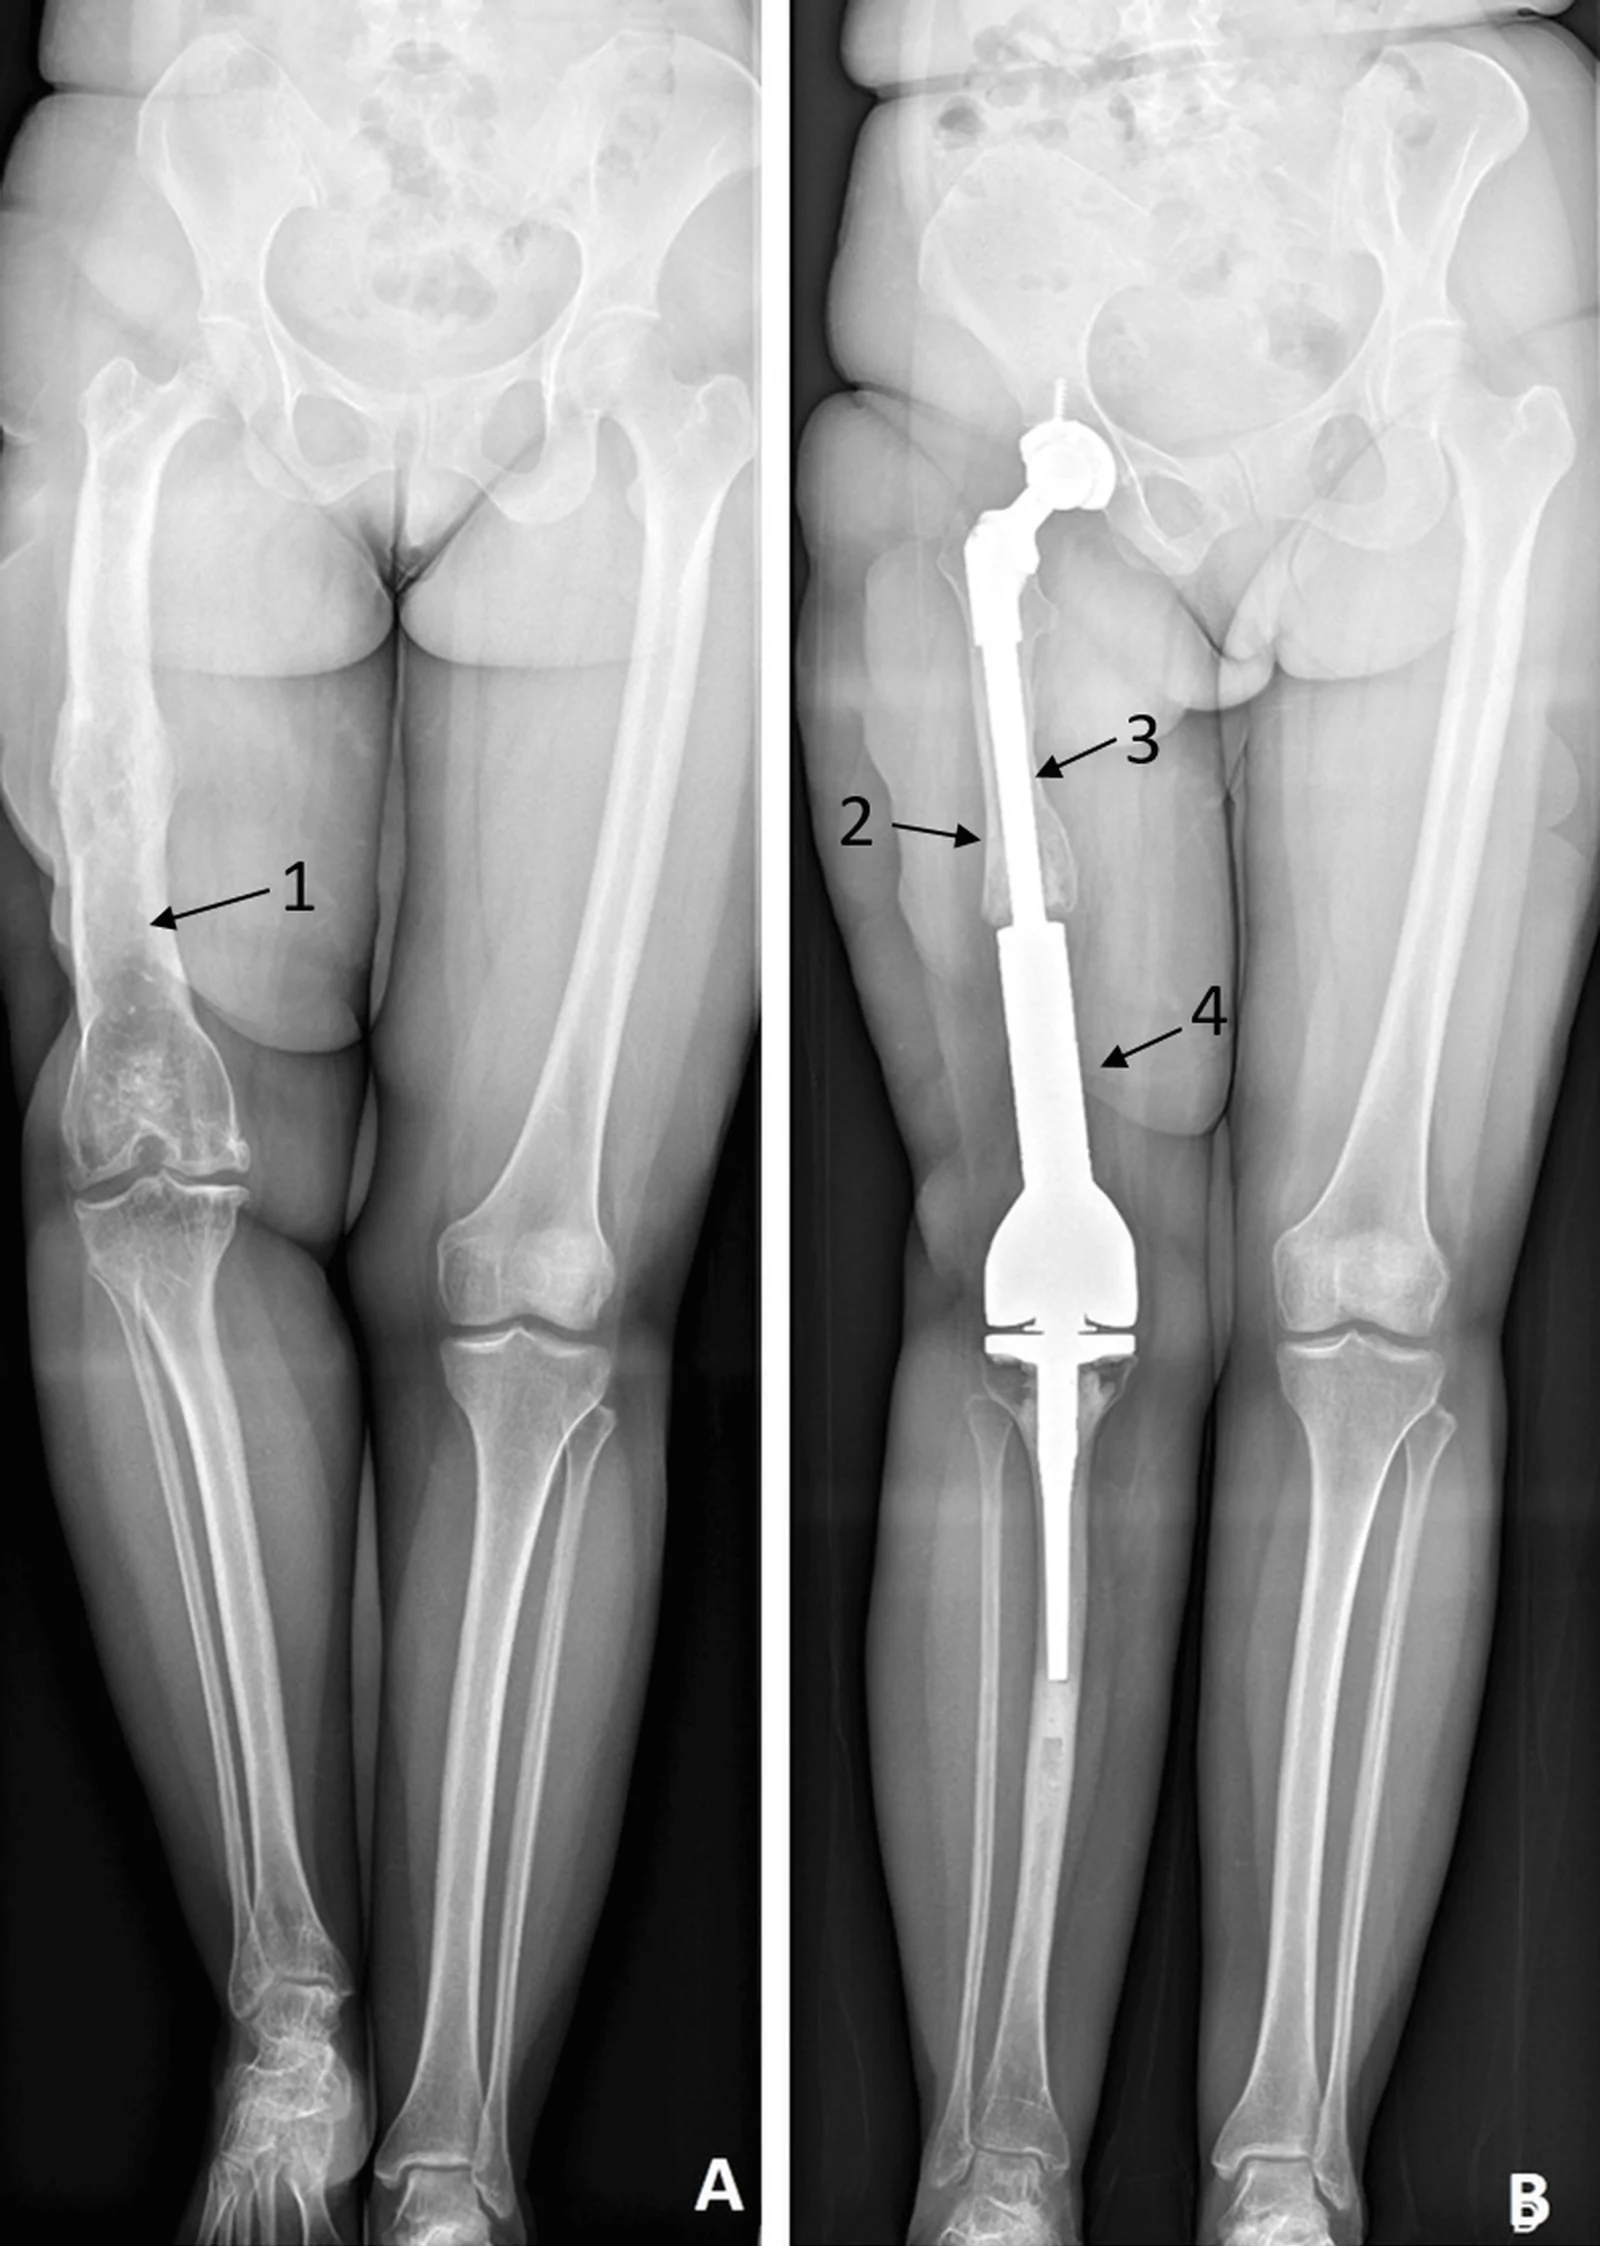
Representative anteroposterior radiographs of a patient treated with a push-through prosthesis for the reconstruction of massive femoral bone loss secondary to chronic osteomyelitis (A) Preoperative radiograph showing extensive femoral bone loss (1). (B) Postoperative radiograph following reconstruction with a push-through prosthesis demonstrating preservation of the residual femoral cortical shell (2), the intramedullary push-through stem traversing the preserved cortical cylinder (3), and the modular distal femoral reconstruction (4)
